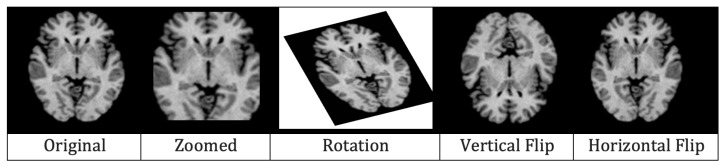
Effects of augmentation on MRI.
